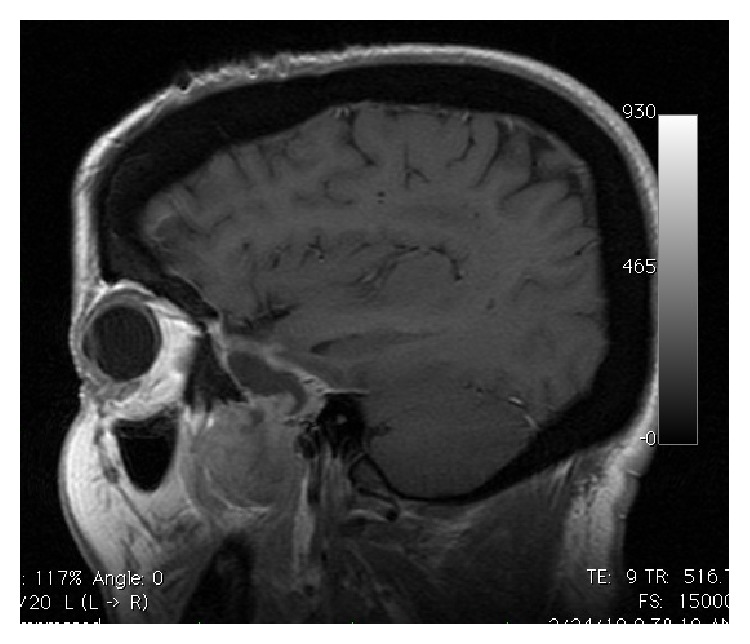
Postoperative (24 hr) follow-up T1-WI parasagittal MRI demonstrates complete resection of tumor from the infratemporal region.
